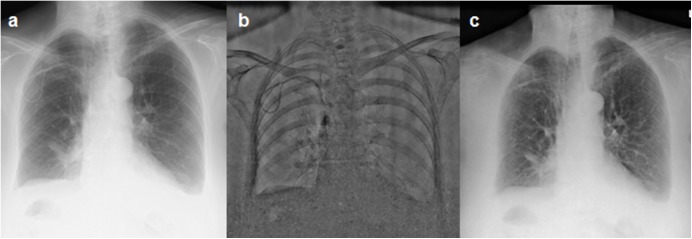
Conventional Radiography and DESR. Conventional radiograph (CR, a). Bone image (b), and soft tissue image (c) of the chest after subtraction of thoracic skeletal structures.
